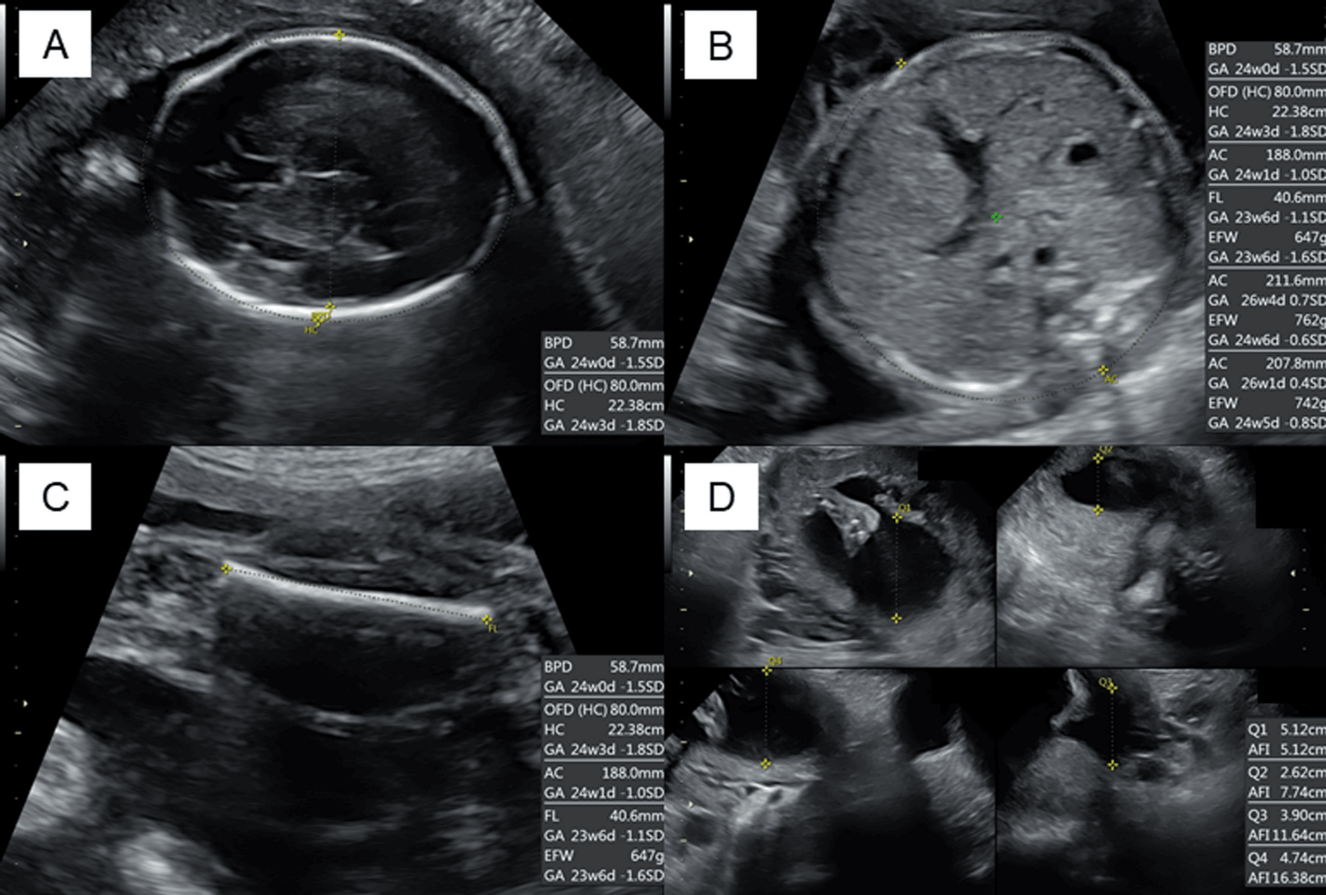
Obstetric ultrasonography (A) The plane used for biparietal diameter measurement, (B) the plane used for abdominal circumference measurement, (C) the plane used for femoral length measurement, and (D) the plane used for amniotic fluid index measurement.
